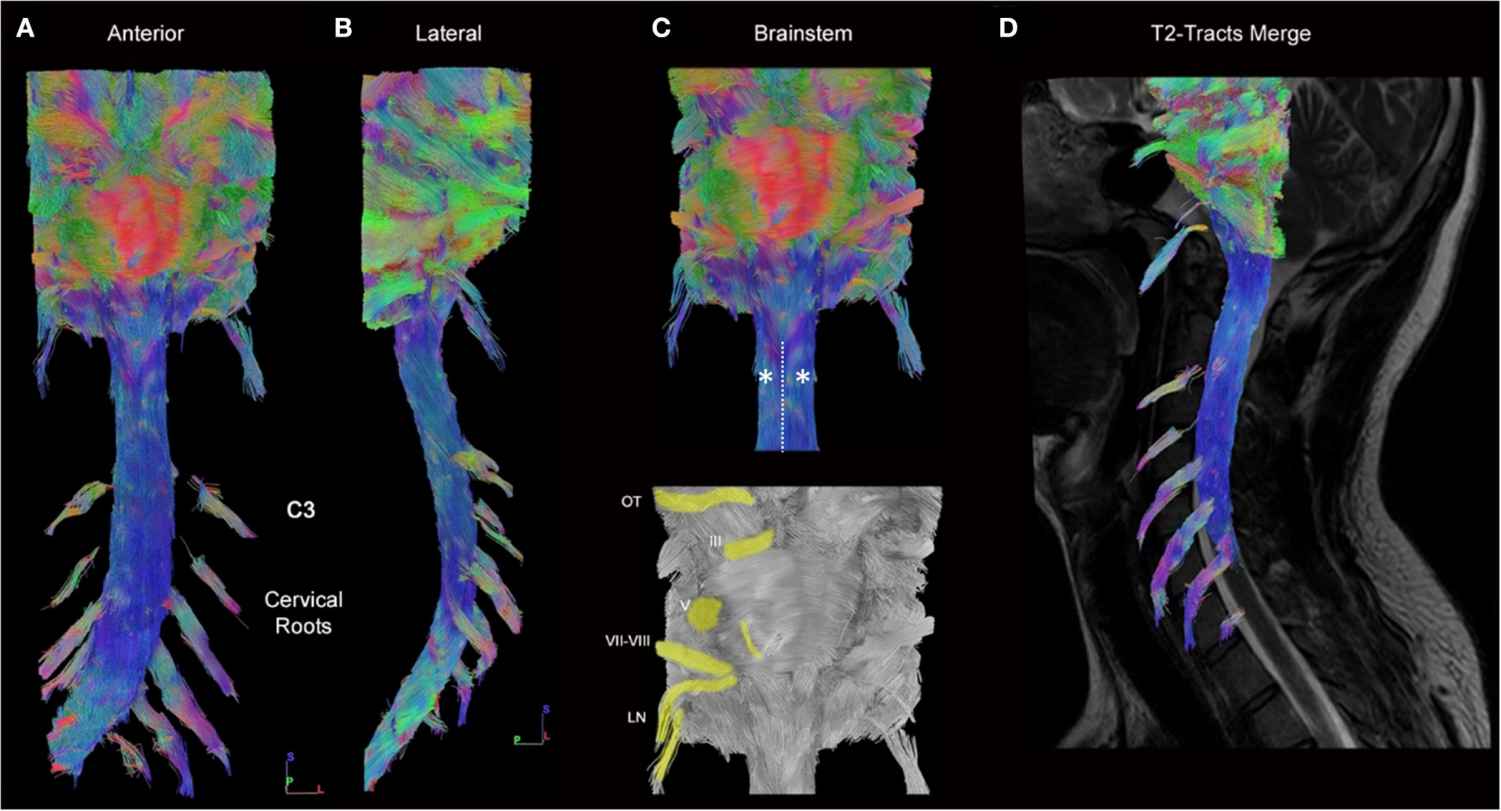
Full cervical cord tractography (healthy subject). (A) Ventral and (B) lateral views of the cervical spinal cord and brainstem tractography showing the ascending/descending pathways with cervical rootlets. The emergence of the rootlets from the spinal cord can be seen laterally (turquoise). Spinal cord fibers (blue) are well reconstructed on the whole length of the spinal cord in a subject who had an important cervical lordosis. (C) From this enlargement of panel A on the brainstem, pontocerebellar fibers are seen (red), cranial nerves (from optic tracts [OT] to lower nerves [LN]), including acoustic-facial bundle [VII–VIII] are highlighted (yellow). The junction between the medulla and spinal cord was identified by its pyramidal bulging (*), and by an invagination that corresponds to the median ventral sulcus (dotted line). (D) The spinal cord tractography is superimposed on the sagittal T2-weighted images.
